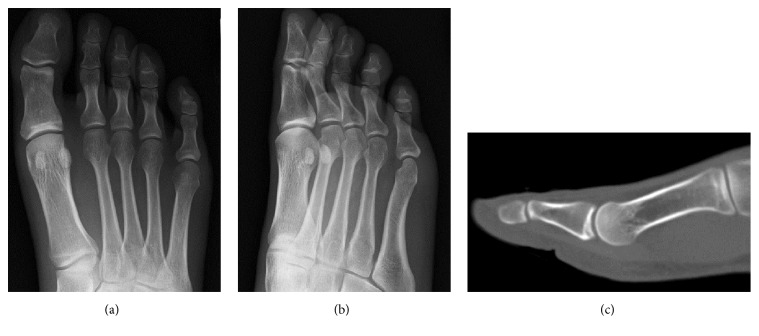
Plain radiographs and computed tomography showed no significant abnormality. (a) Anteroposterior radiograph of the foot. (b) Oblique radiograph of the foot. (c) Sagittal plane of the great toe in computed tomography.
